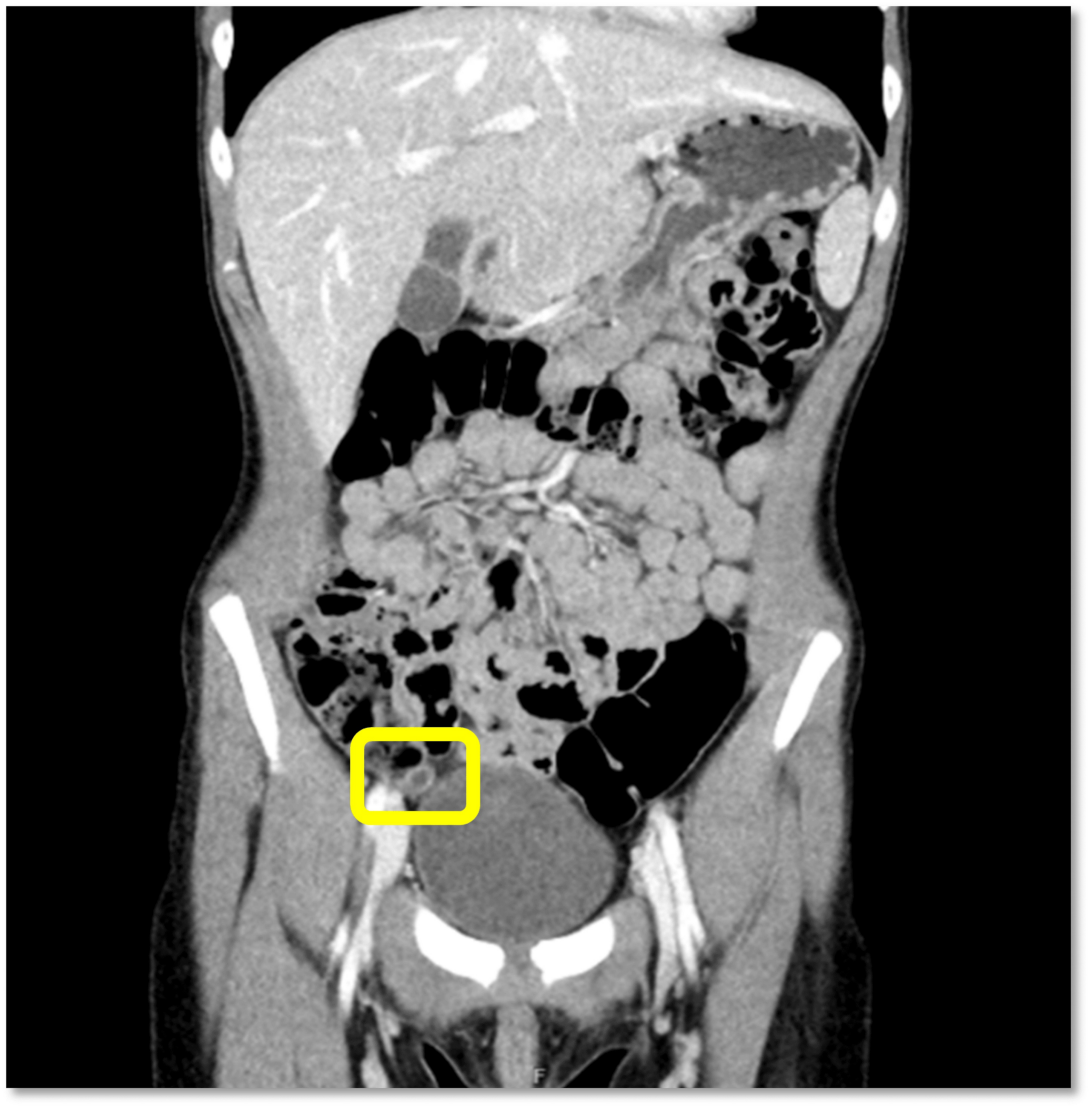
A coronal computed tomography (CT) image of the abdomen and pelvis with intravenous contrast demonstrating a mildly distended appendix. The appendix (highlighted in a yellow box) is mildly distended, measuring up to 0.7-0.8 cm in diameter, and contains both fluid and air. The appendiceal wall demonstrates borderline mucosal thickening without peri-appendiceal fat stranding or surrounding fluid collections. No intraluminal calcifications or appendicoliths are seen. Findings are consistent with early acute appendicitis.
